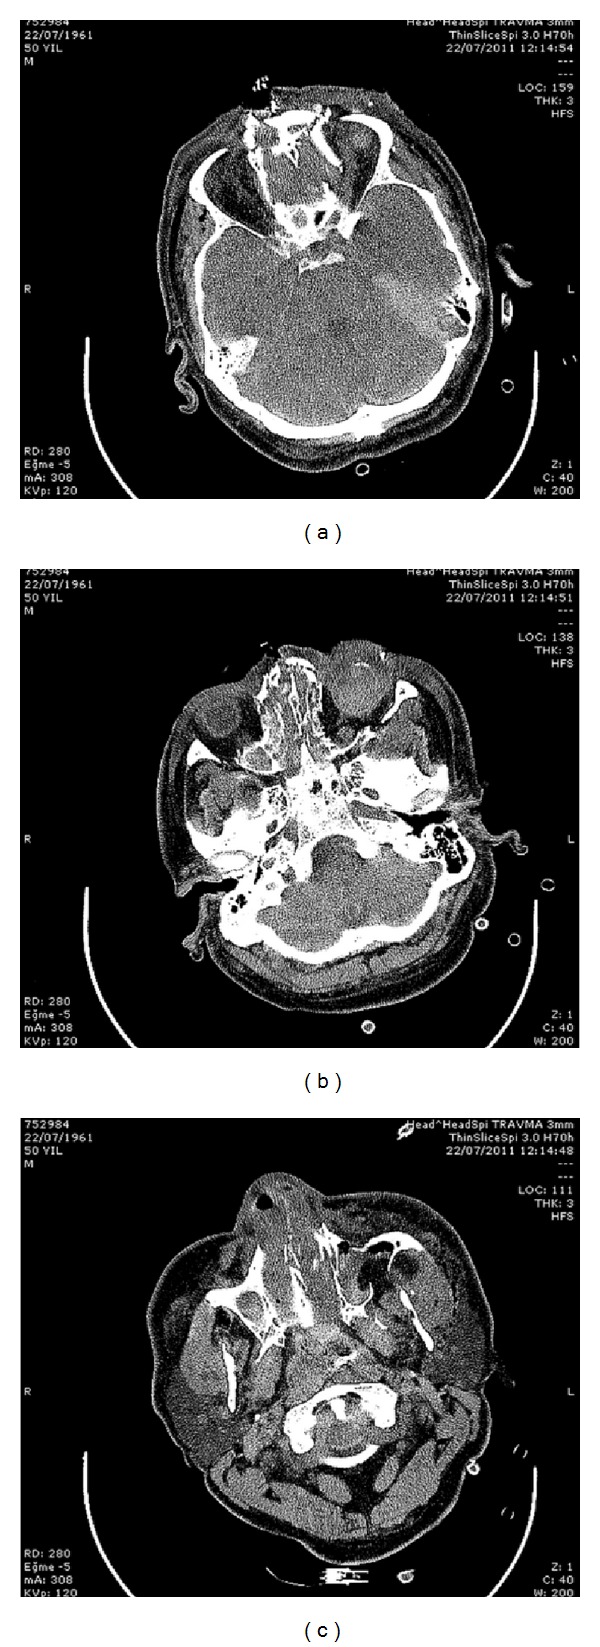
Head CT showing multiple fractures of the face. The patient suffered from depression fracture in the frontal (a), orbital and nasal (b), and maxillary (c) bones.
